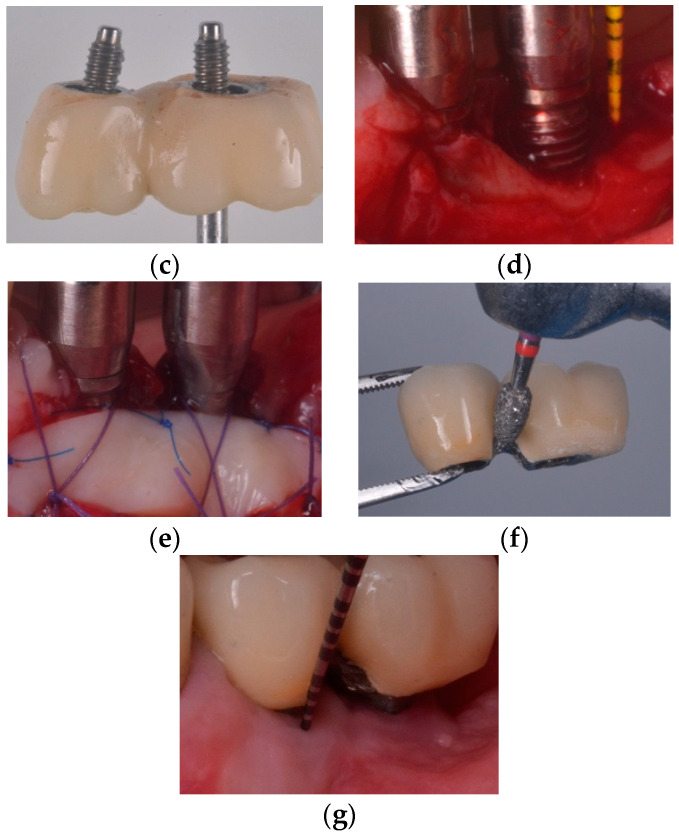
(a) Clinical presentation of peri-implantitis; (b) Radiographic image compatible showing moderate (<50%) bone loss; (c) Inadequate prosthesis emergence profile; (d) Partial-thickness apical position flap; (e) Soft tissue conditioning by means of free epithelial graft; (f) Prosthesis contour modification to facilitate proximal access during self-performed oral hygiene; (g) Clinical resolution of peri-implantitis associated with a gain of keratinized mucosa.
